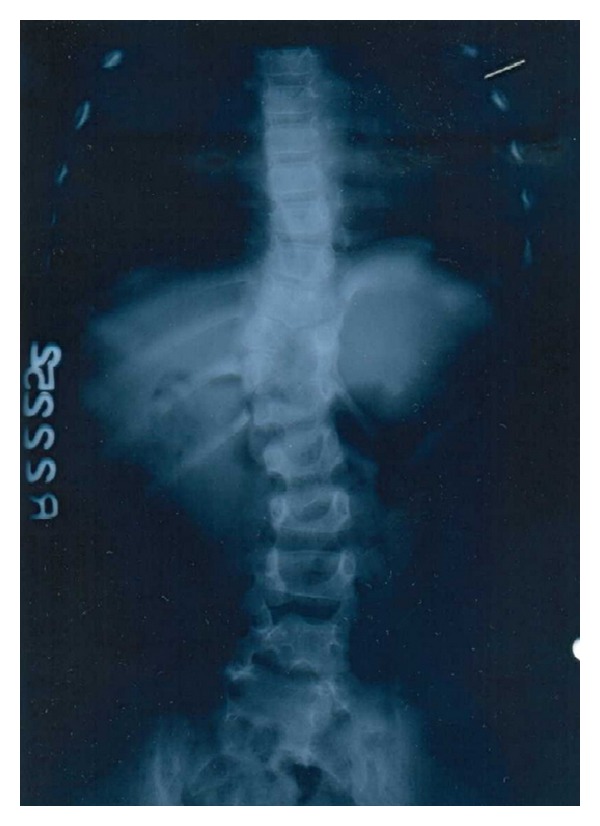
X-ray of the chest region and abdomen showing absence of lower ribs on the left side and scoliosis.
